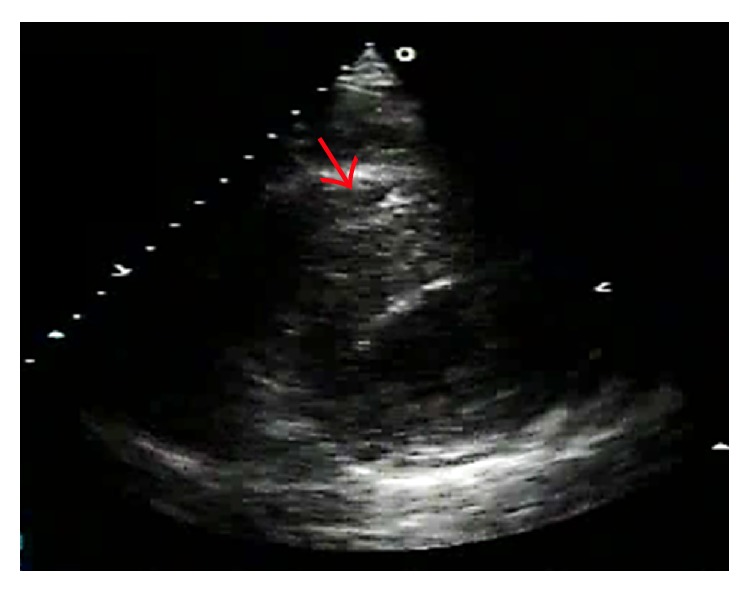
Increased interventricular septum thickness and abnormal motion by postprocedural echocardiography.
